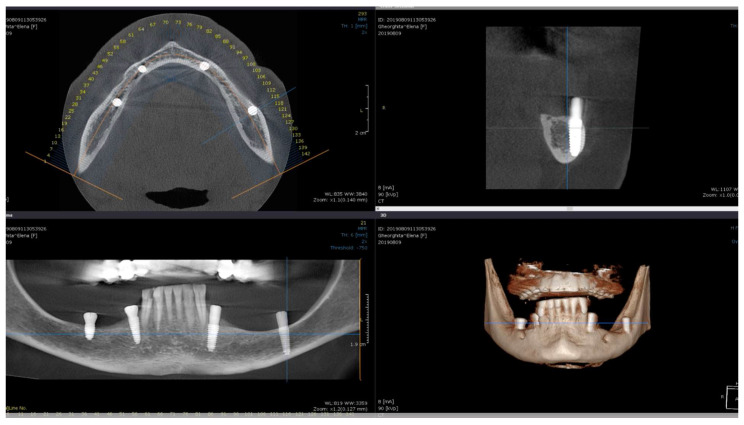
Postoperative CBCT of patient no. 6: (Upper left)—axial view of the mandible; (upper right)—sagittal left view of the buccally tilted implant (37); (lower left)—coronal view of the mandible; (lower right)—3D view of the mandible.
